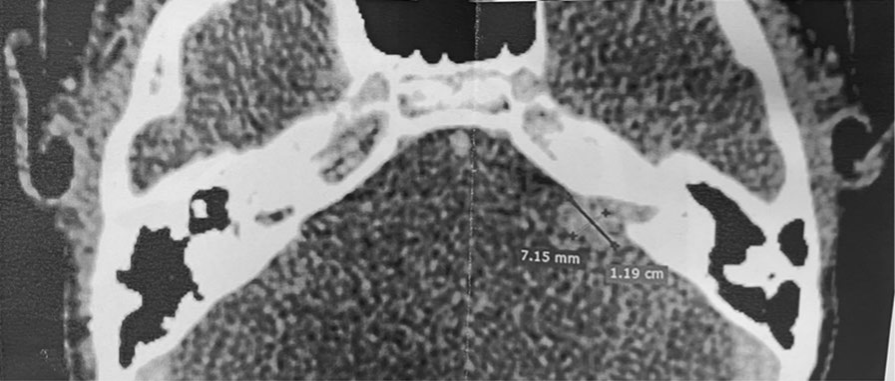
Axial contrast CT images. A contrast-enhancing mass is clear in the left CPA and IAC
